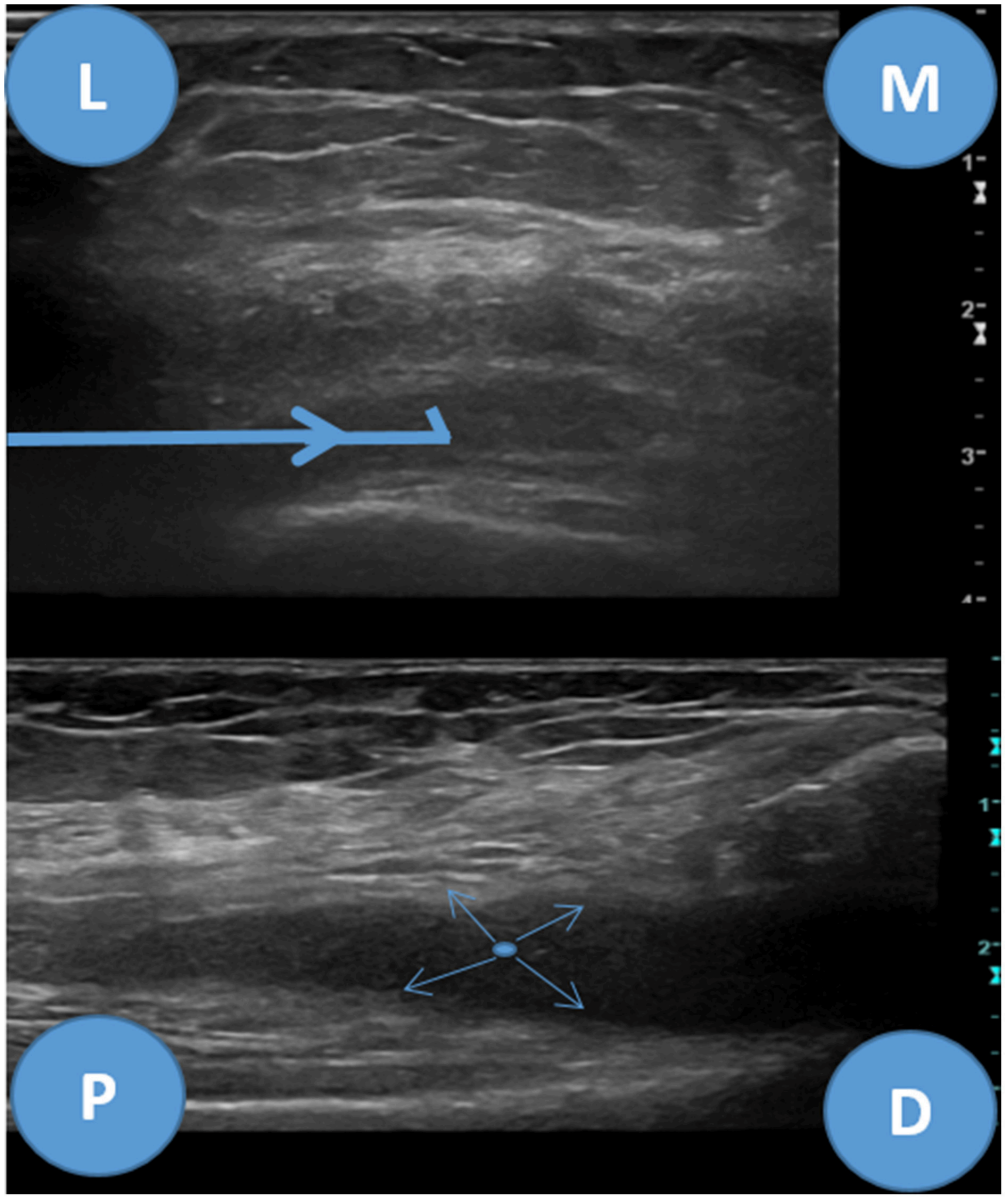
Superolateral approach for knee biopsy, ultrasound images. Upper image—transverse view of the suprapatellar pouch of the knee joint, L, lateral; M, medial. Bold blue arrow—biopsy needle trajectory. Lower image—longitudinal view, P, proximal; D, distal. Thin blue arrows—needle throw directions.
